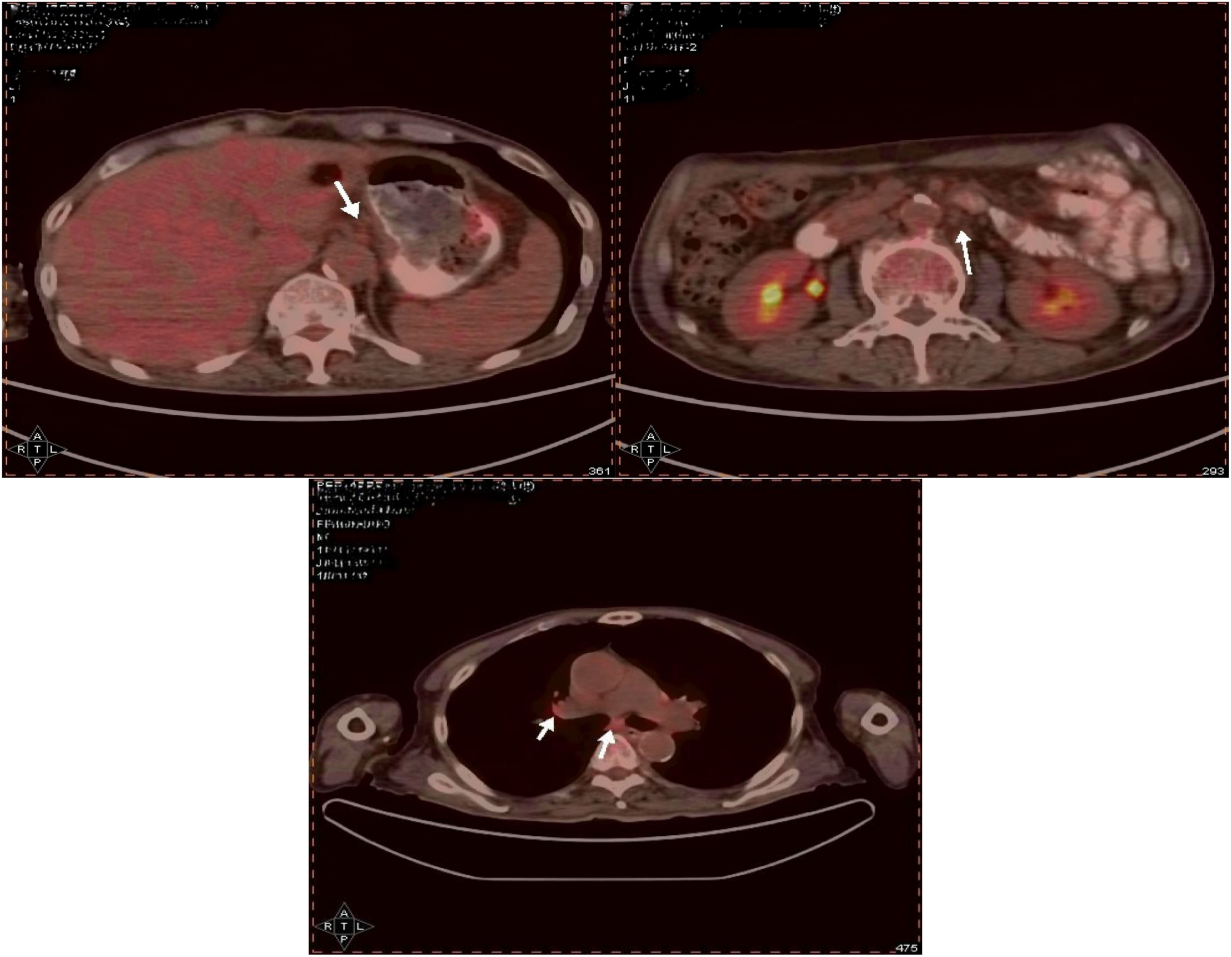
The gastric wall, liver, and lymph nodes show no increased FDG metabolism, suggesting that tumor activity is suppressed.
